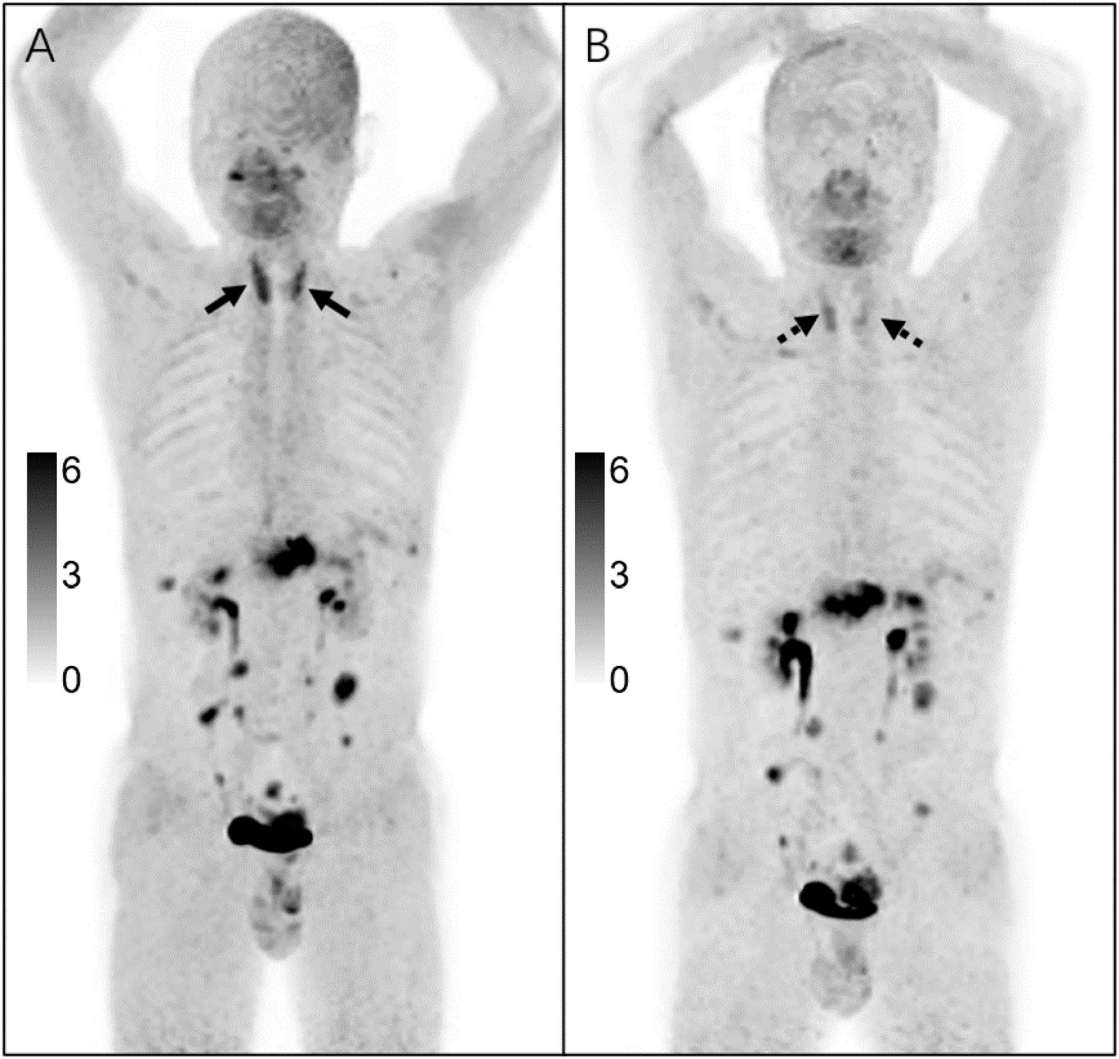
A 58-year-old man was diagnosed with peritoneal metastasis due to post-operative re-examination of pancreatic cancer. After receiving Carrelizumab immunosuppressive therapy, ^68^Ga-FAPI PET/CT was performed [(A): after 1 cycle; (B): after 4 cycles]. After the first cycle, the thyroid showed diffuse increased FAPI uptake with SUVmax of 6.1, and TBR 7.46; blood analysis showed FT3 2.86, FT4 1.18, TSH 13.193, TgAb 12.06, and TPOAb <0.05; after the fourth cycle, the thyroid also showed diffuse increased FAPI uptake with SUVmax of 3.9, and TBR 6.26; blood analysis showed FT3 2.49, FT4 1.02, TSH 7.096, TgAb <0.12, and TPOAb <0.05; Finally, a diagnosis of immune-related thyroiditis was made.
